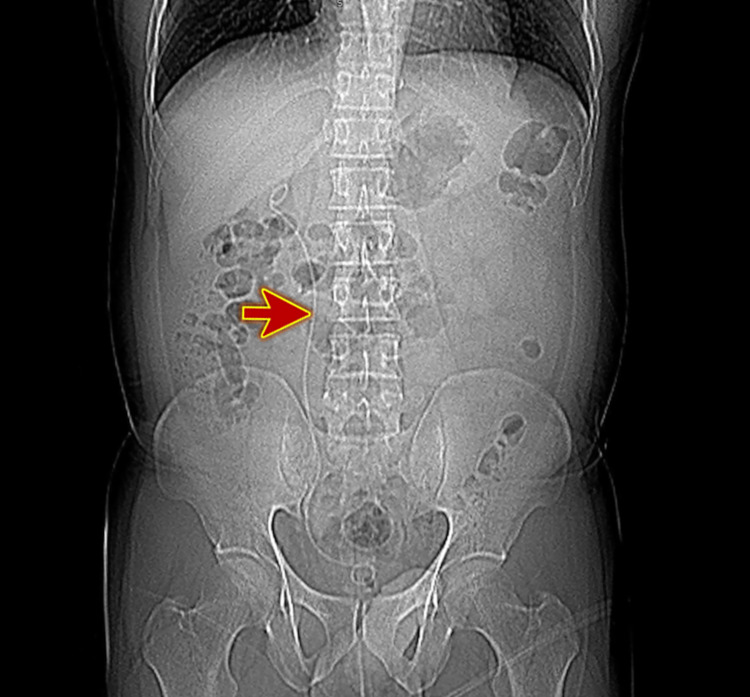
KUB X-ray (scout view) showing the stent in position (red arrow). KUB, kidney, ureter, and bladder
